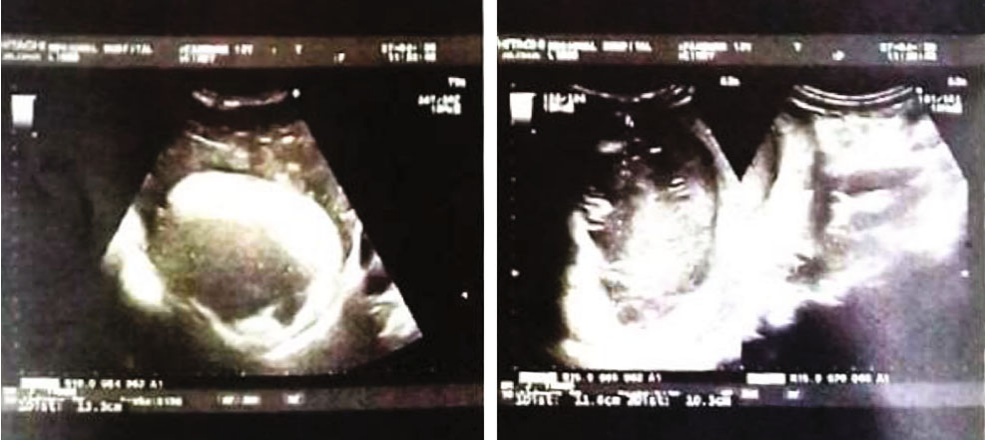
The ultrasound reveals a giant heterogenous mass with thick walls and air bubbles within the right lower lobe of the liver measuring 141 mm suggestive of an amoebic liver abscess.
